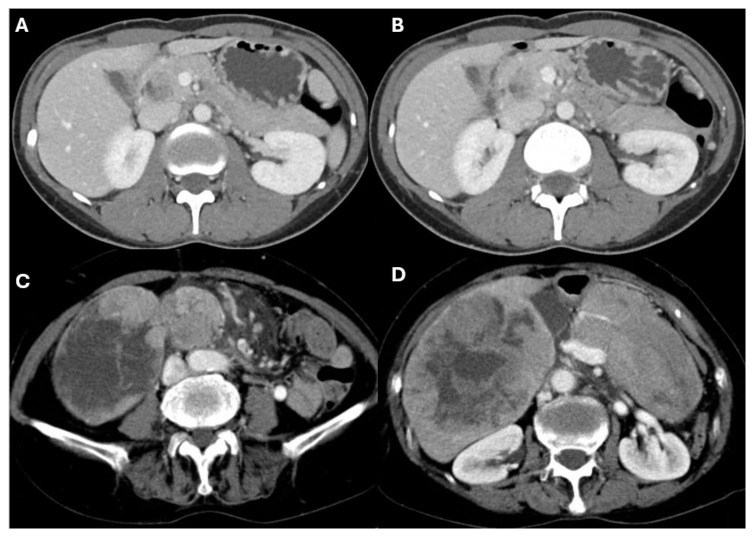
Computed tomography (CT) findings of pancreatic acinar cell carcinoma. (A,B) CT images in the arterial phases reveal a relatively well-defined, hypo-enhancing heterogenous solid mass in the pancreatic head/body without associated pancreatic duct dilation. (C) A large, heterogeneous mass is observed in the hepatic parenchyma, with internal low-density areas suggestive of central necrosis or cystic degeneration, accompanied by a huge mass in the pancreas head showing mixed enhancement features. (D) A mixed solid and cystic tumor replaces the right hepatic parenchyma, and a poorly defined tumor with necrotic components replaces a significant portion of the pancreatic body and tail.
